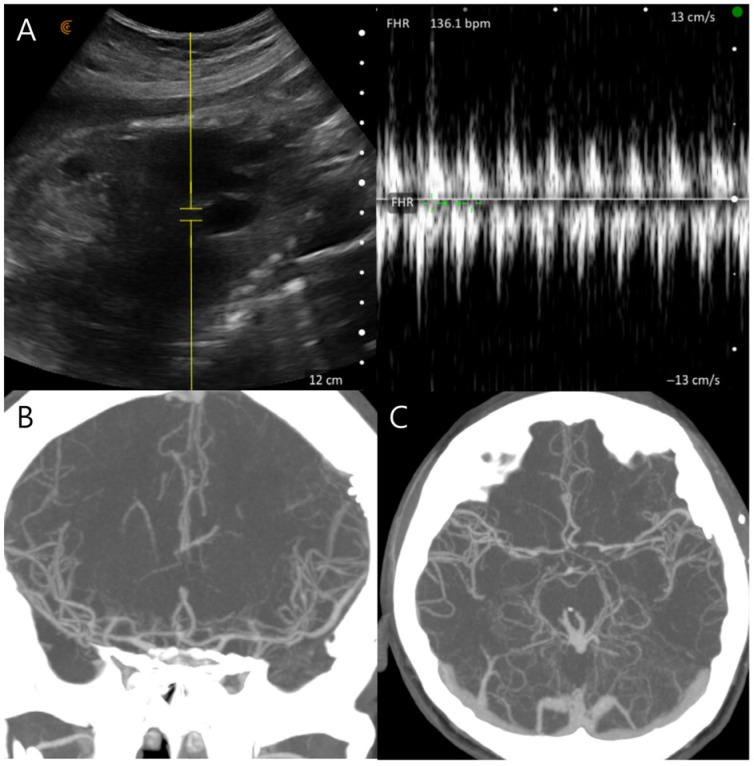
(A) The fetus remained reactive after chemical angioplasty. The yellow area represents the sample region used for Doppler flow analysis. (B,C) Follow-up CT angiography, performed 2 days after chemical angioplasty, reveals further improvement of the vasospasm.
